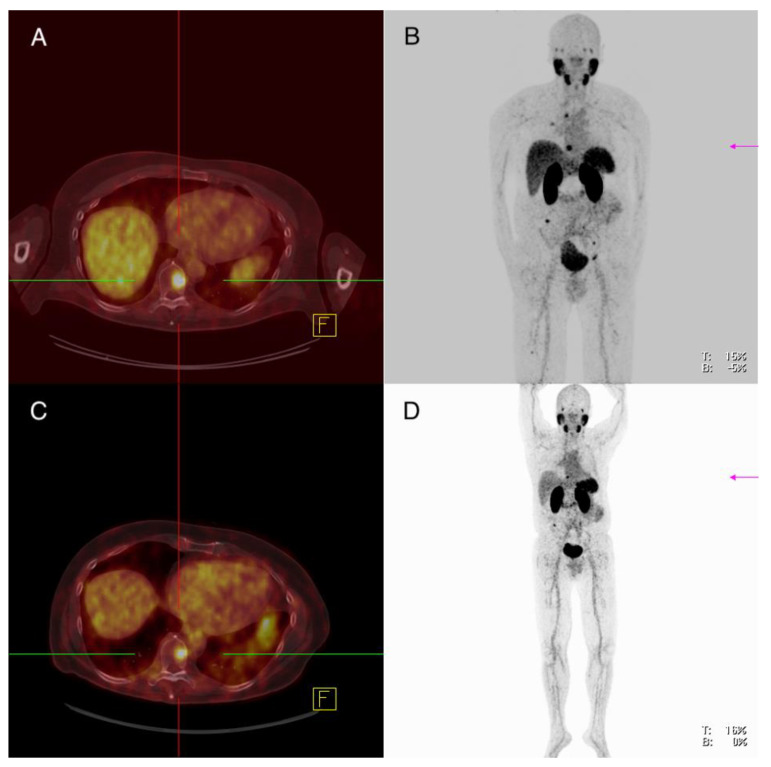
Patient 8. Second ^68^Ga-PMSA-PET/CT (A,B), first ^68^Ga-PMSA-PET/CT (C,D). Second ^68^Ga-PSMA-PET/CT: Progressive disease with size progression of known osseous lesions and multiple new osseous lesions with increased PSMA expression.
